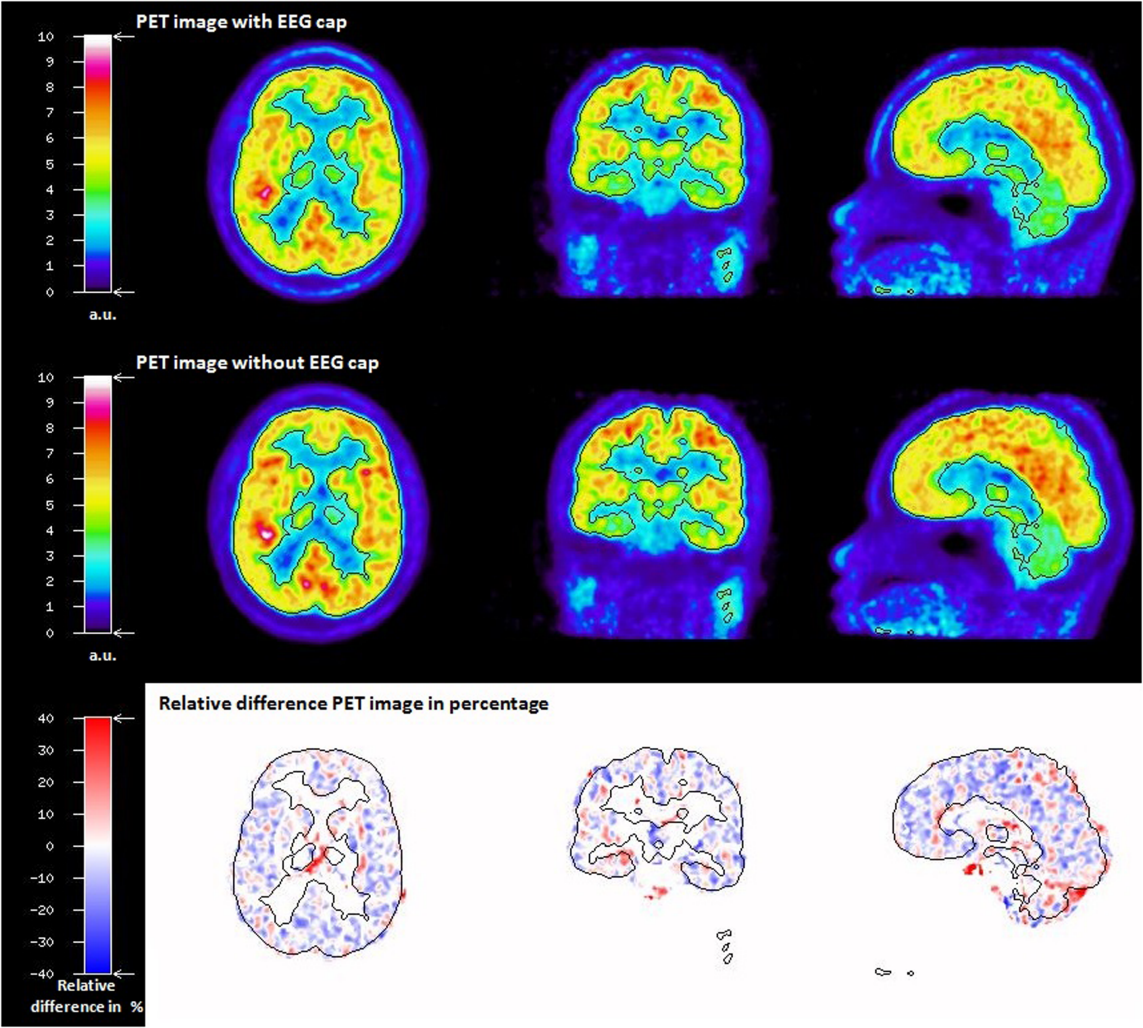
FDG PET SUV image of a human subject with EEG cap (top row) and without EEG cap (middle row), both reconstructed with an AC map without EEG cap; relative difference image in percentage between the above FDG PET images (bottom row).
